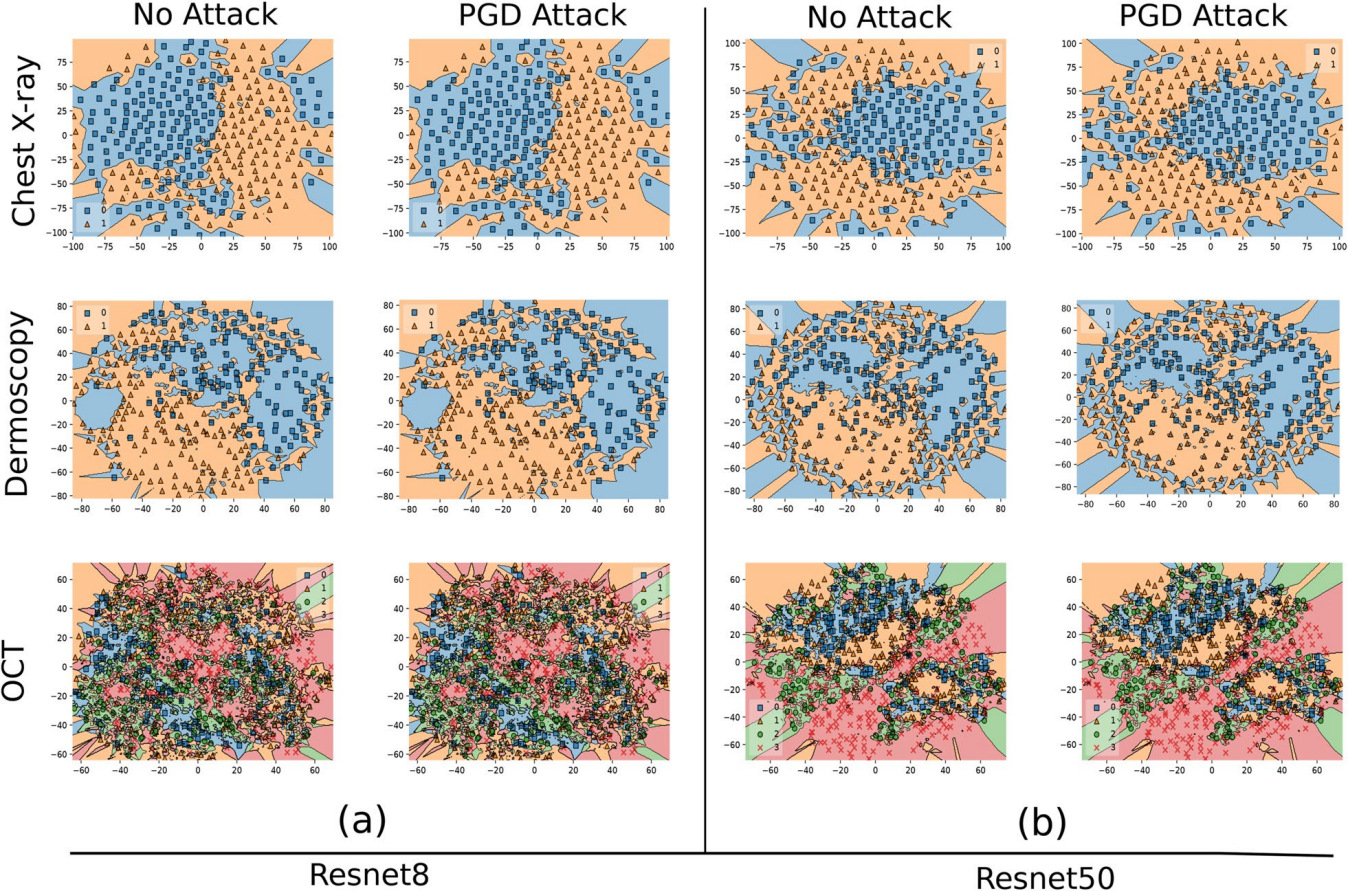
Decision boundary projection of adversarially trained (AT) Resnet8 and Resnet50 for Chest X-ray, Dermoscopy & OCT datasets. Unperturbed samples were projected on column 1 (no attack) and perturbed samples were projected on column 2 (PGD attack) for (a, b), respectively. The complexity of adversarial trained decision boundaries increased compared to the corresponding standard trained model decision boundary in Fig. 5
